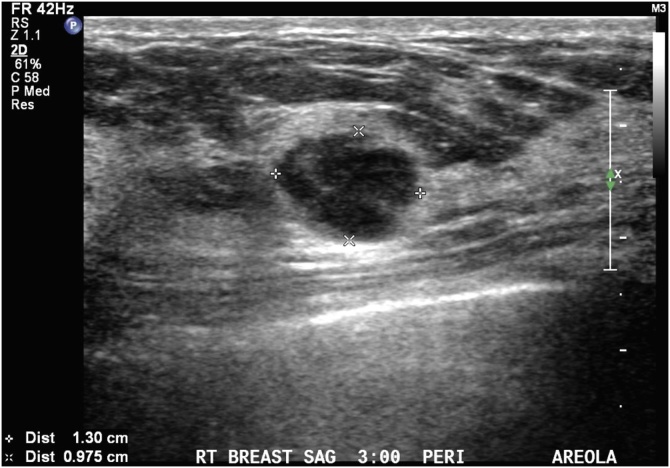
Ultrasound of right breast showing a heterogeneous, hypoechoic nodule.
